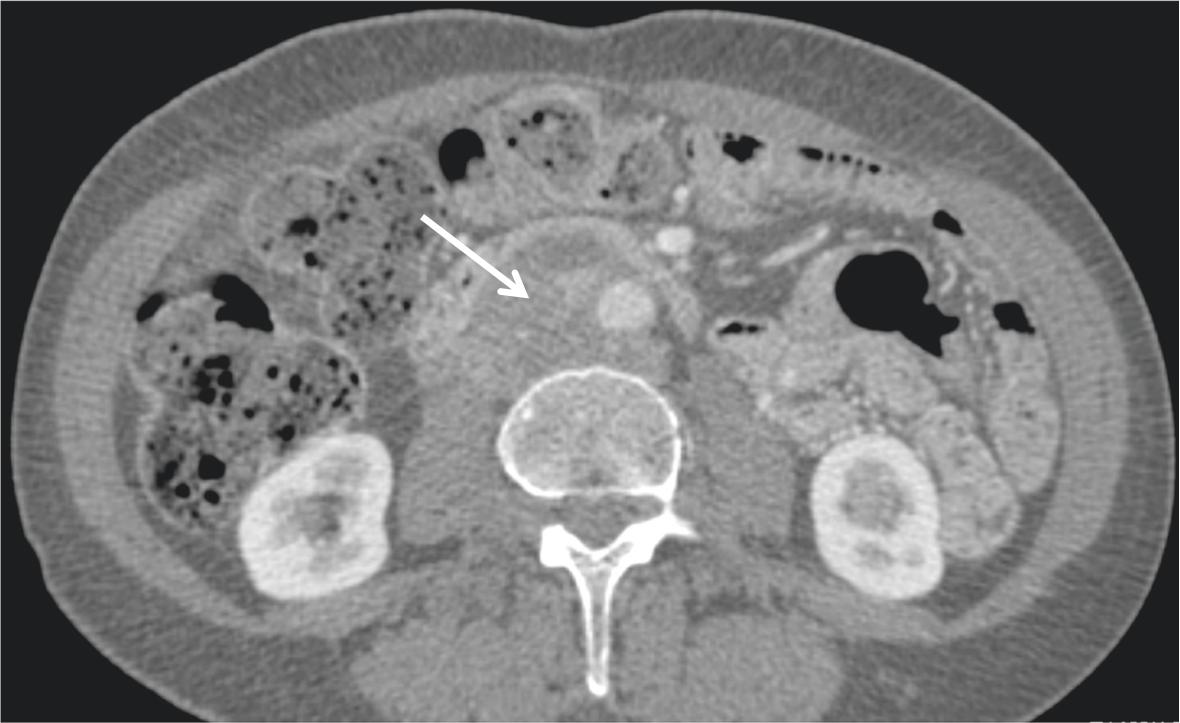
CT scan image. Axial view showing retroperitoneal heterogeneous soft tissue density mass that compresses and thrombosis the infrarenal IVC (white arrow).
